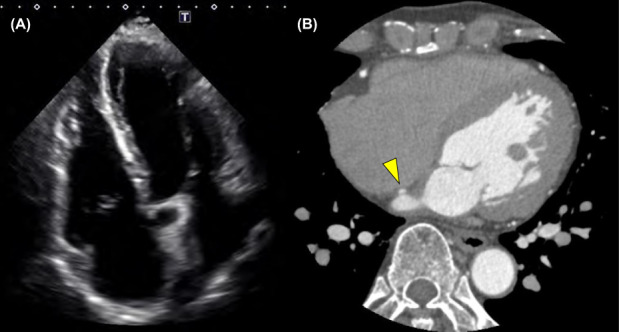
Transthoracic echocardiography image of the dilated left atrium and right ventricle. (A) Dilation of the left atrium and right ventricle. Slightly impaired right ventricular systolic function (left atrial dimension, 41.4 mm; left atrial volume, 58.2 mL; left ventricular ejection fraction, 57.9%; basal right ventricular dimension, 49.4 mm; mid‐right ventricular dimension, 33.5 mm; longitudinal right ventricular dimension, 106.2 mm; and fraction area change of the right ventricle, 30.7%) was also noted. The right ventricle dilation is consistent with the history of an atrial septal defect (ASD). No leakage was identified around the patch. (B) Coronary angiography CT performed 7 years previous revealed contrast leakage from the left to the right atrium, suggestive of a fistula (arrowhead).
